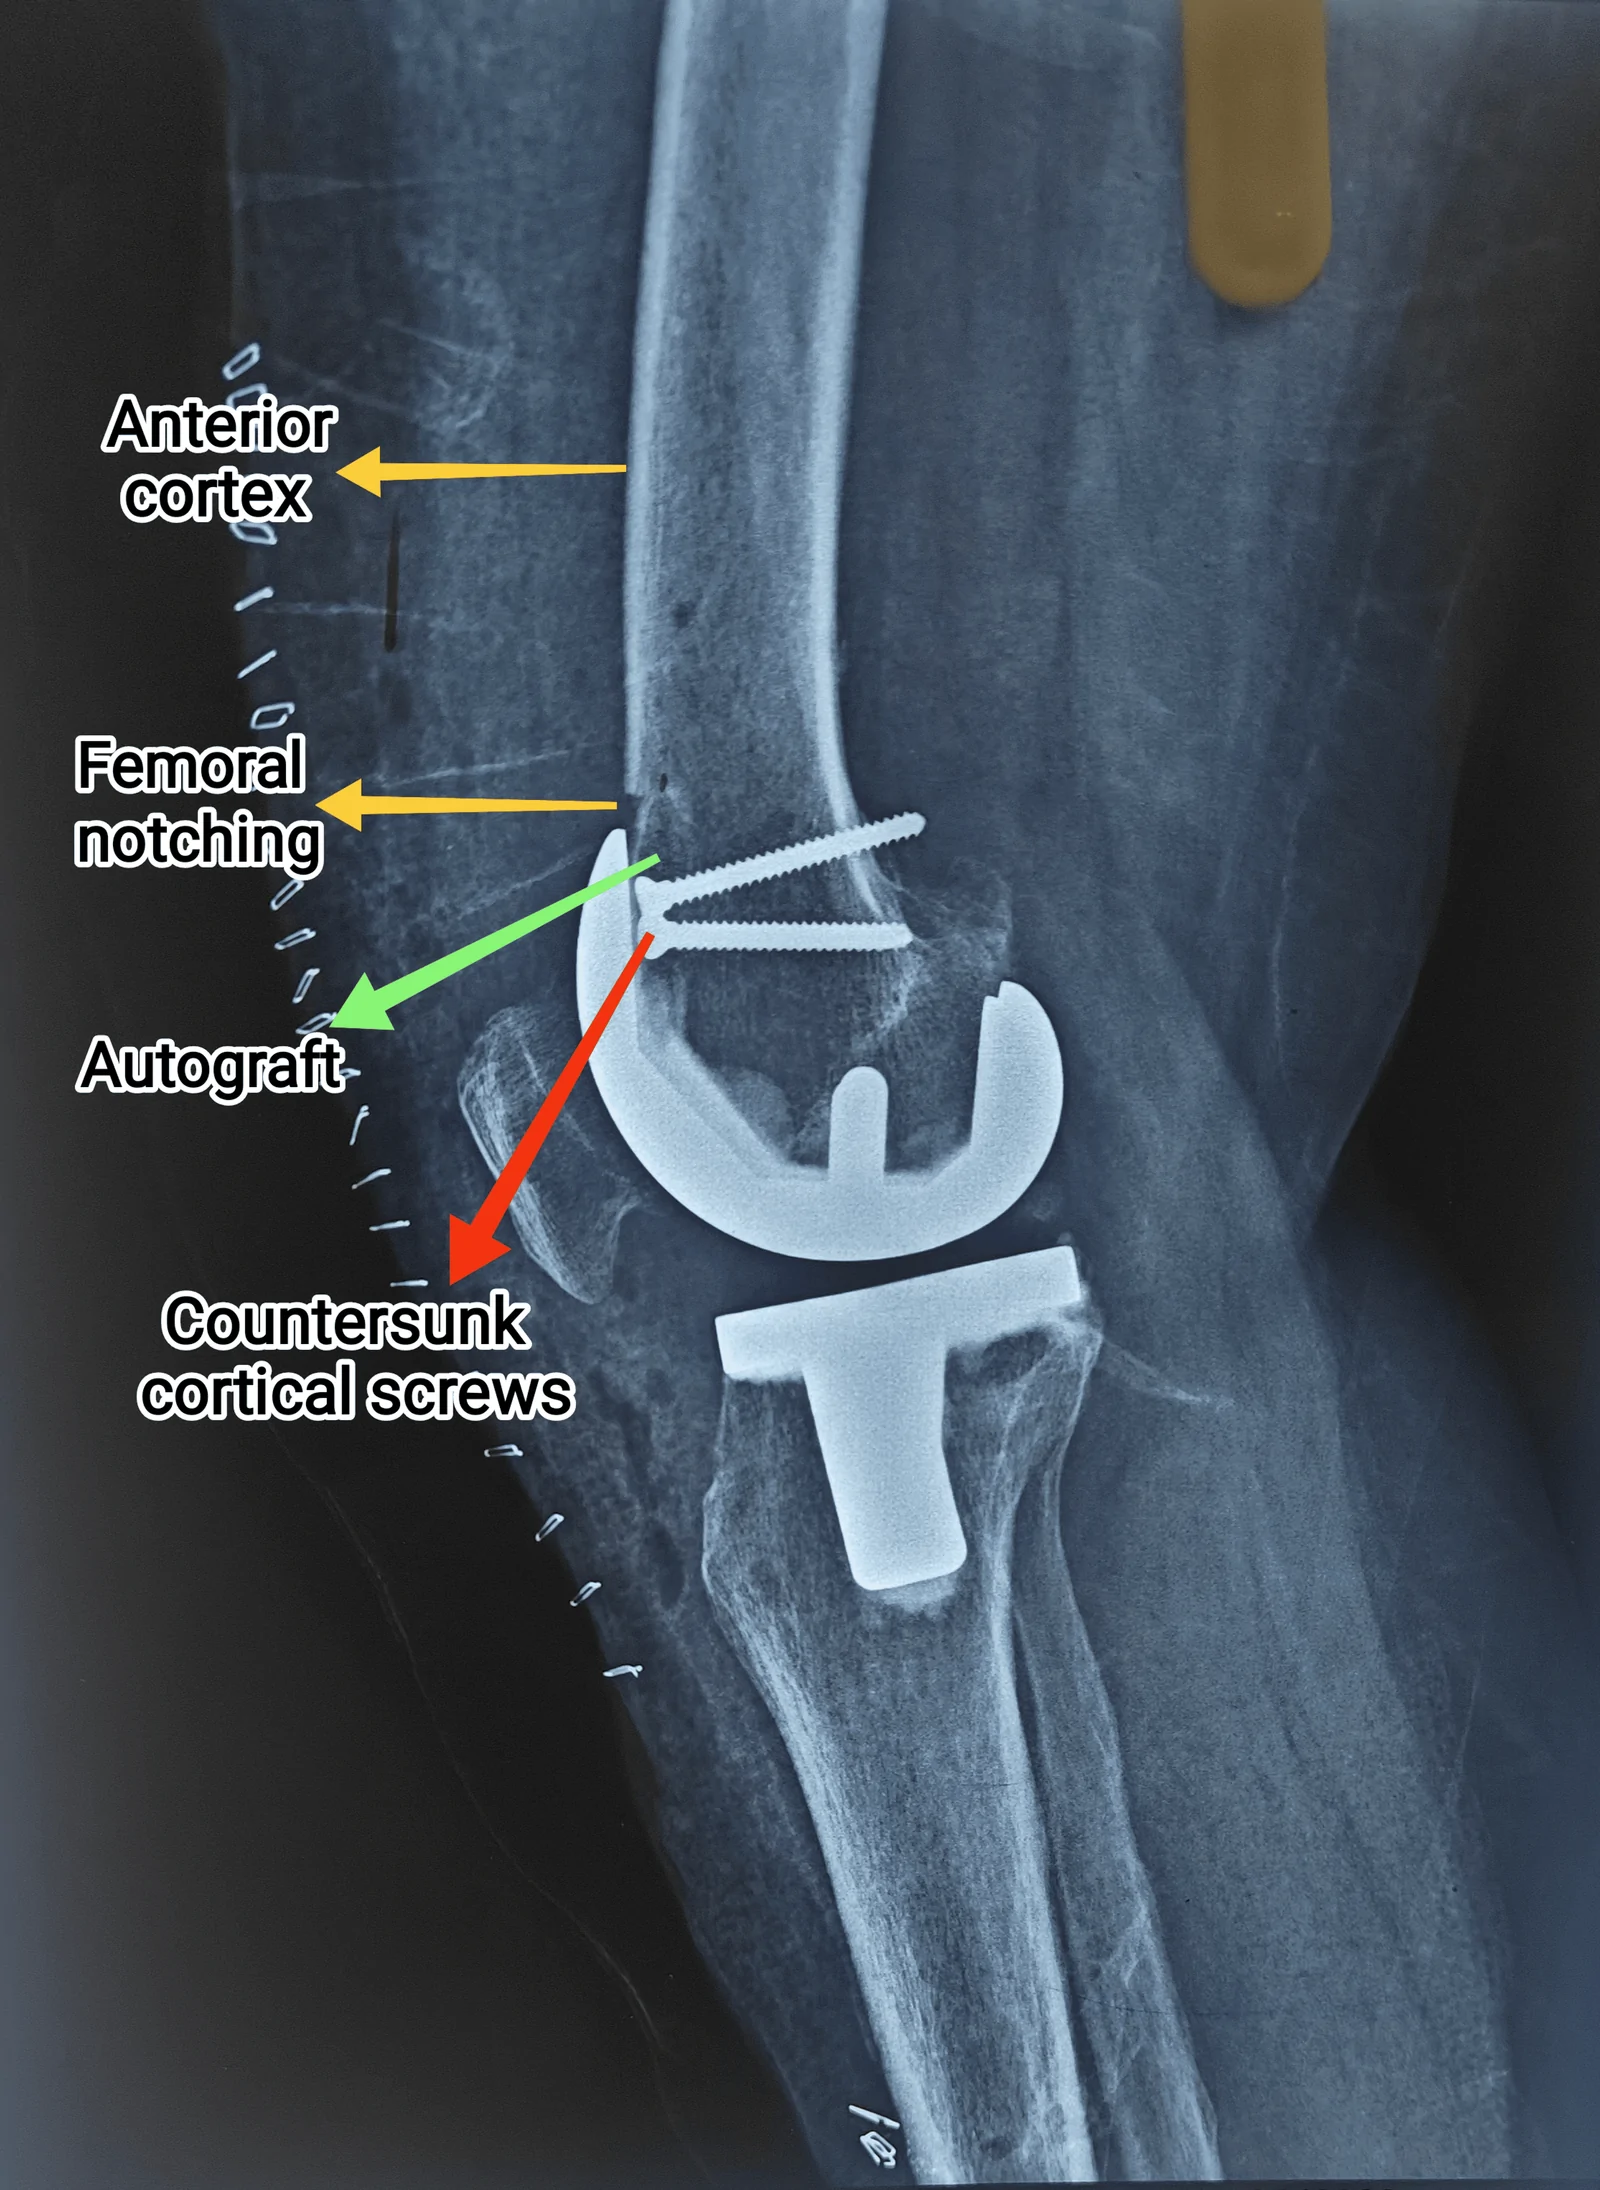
Immediate postoperative lateral-view radiograph of the right knee demonstrating Grade III femoral notching reconstructed using a structural proximal tibial cut autograft, fixed with two countersunk cortical screws, with standard cruciate-retaining primary femoral and tibial components in situ.
